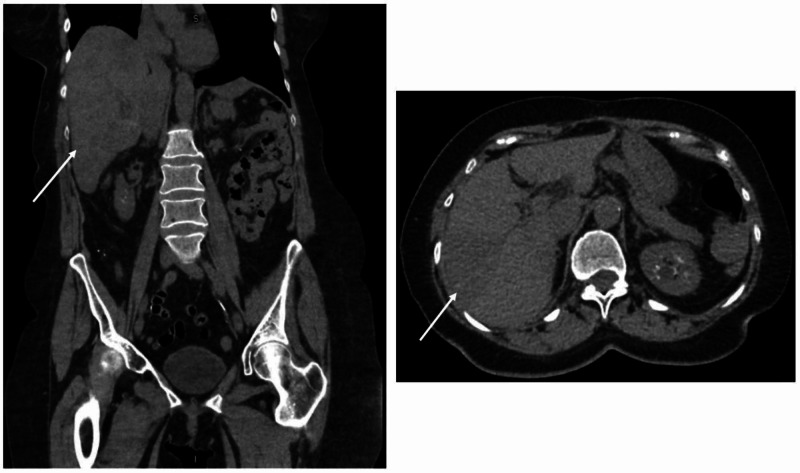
CT abdomen, pelvis: re-evaluation for metastatic disease Significant for hepatomegaly, with multiple areas of hypodensity concerning for metastasis Right) Coronal view; Left) Axial view CT: computed tomography
